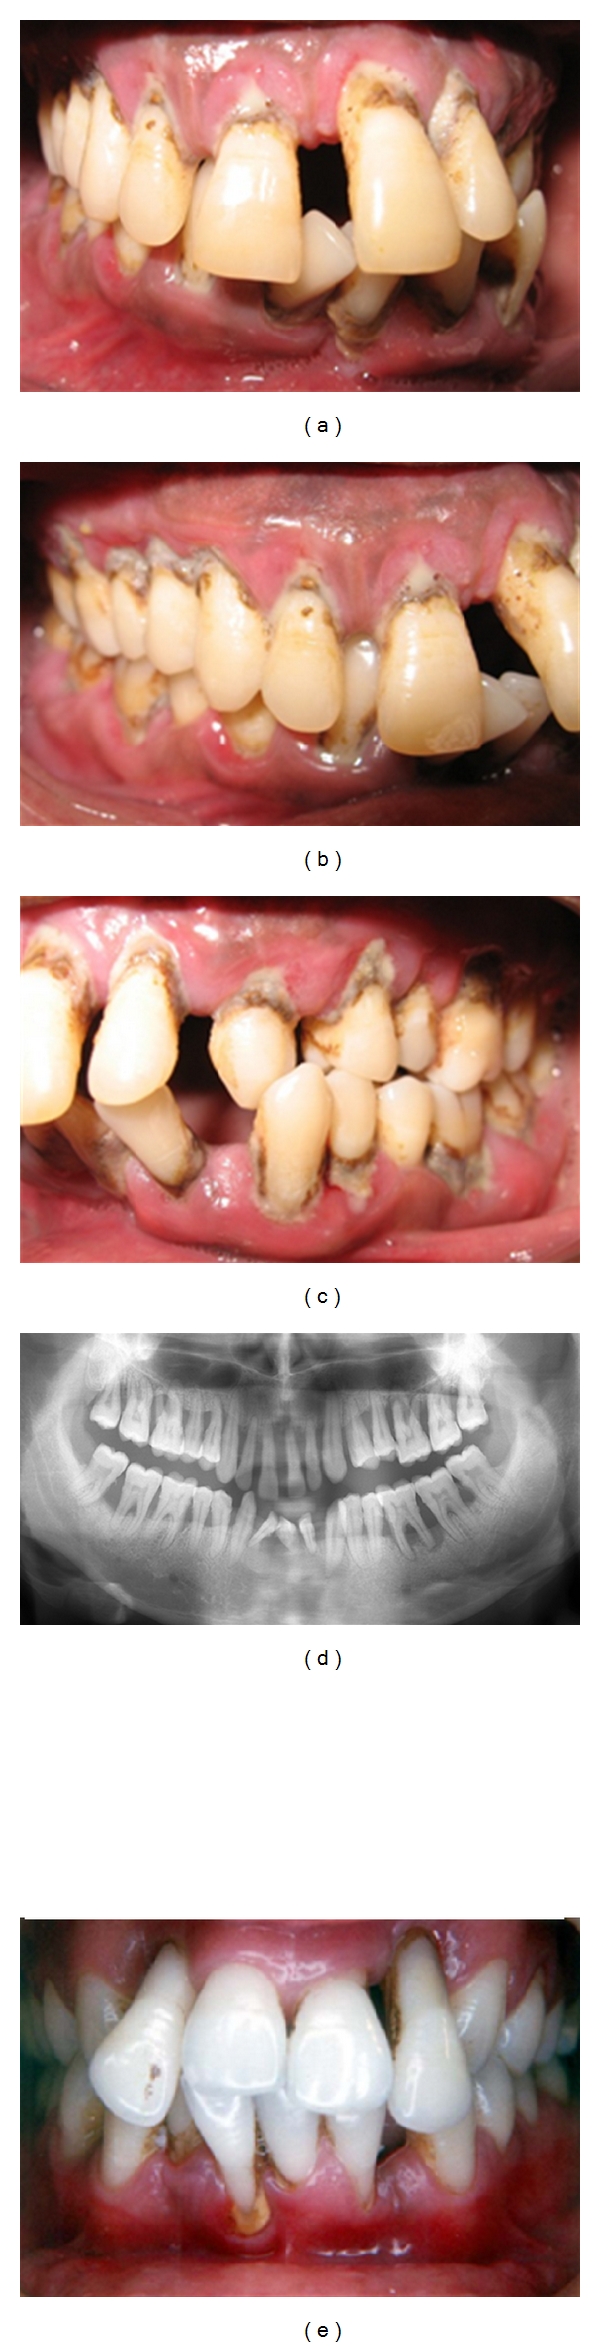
(a)–(d) Clinical and radiographic appearance of GAgP in a 25-year-old smoker with poor oral hygiene. (e) A 19-year-old smoker with GAgP demonstrating severe periodontal destruction.
